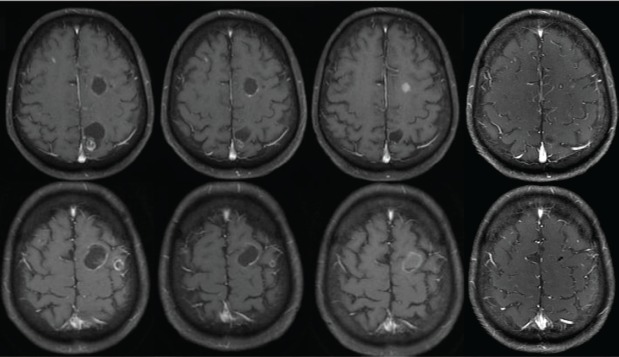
MRI in axial T1-weighted post contrast images demonstrates cystic lesions with ring enhancement in the baseline examination (A and B), delineating cystic areas and ill-defined nodular areas within it, with no surrounding oedema. There was a slight reduction of the lesions after 3 months of ceritinib (C and D), with signs of a more evident response after 6 months (E and F), with reduction of the cystic component of some lesions, and after 9 months (G and H), with reduction of the cystic components and the enhancement.
